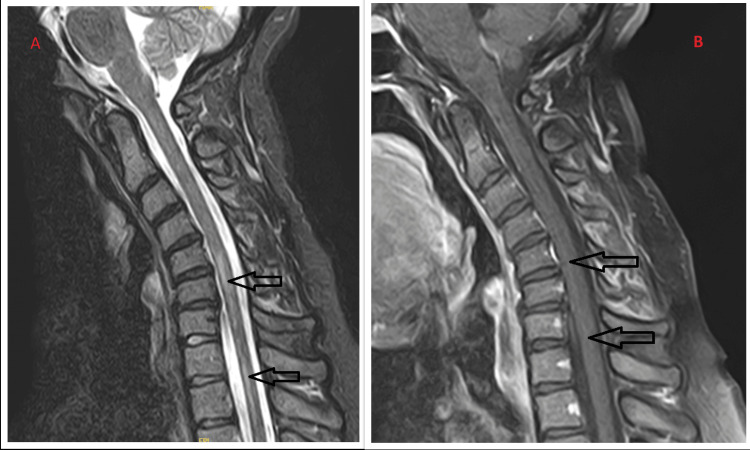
C-spine MRI (on presentation) 1A. Sagittal C-spine MRI with increased T2/STIR cord signal extending longitudinally from C5-C7 and C8-T1 (black arrows). Reversal of the anatomical cervical lordosis. Degenerative changes and disc bulges from C4-C7. 1B. Postcontrast T1 sagittal images revealed no abnormal enhancement within the cervical cord. C-spine: cervical-spine; STIR: short tau inversion recovery
